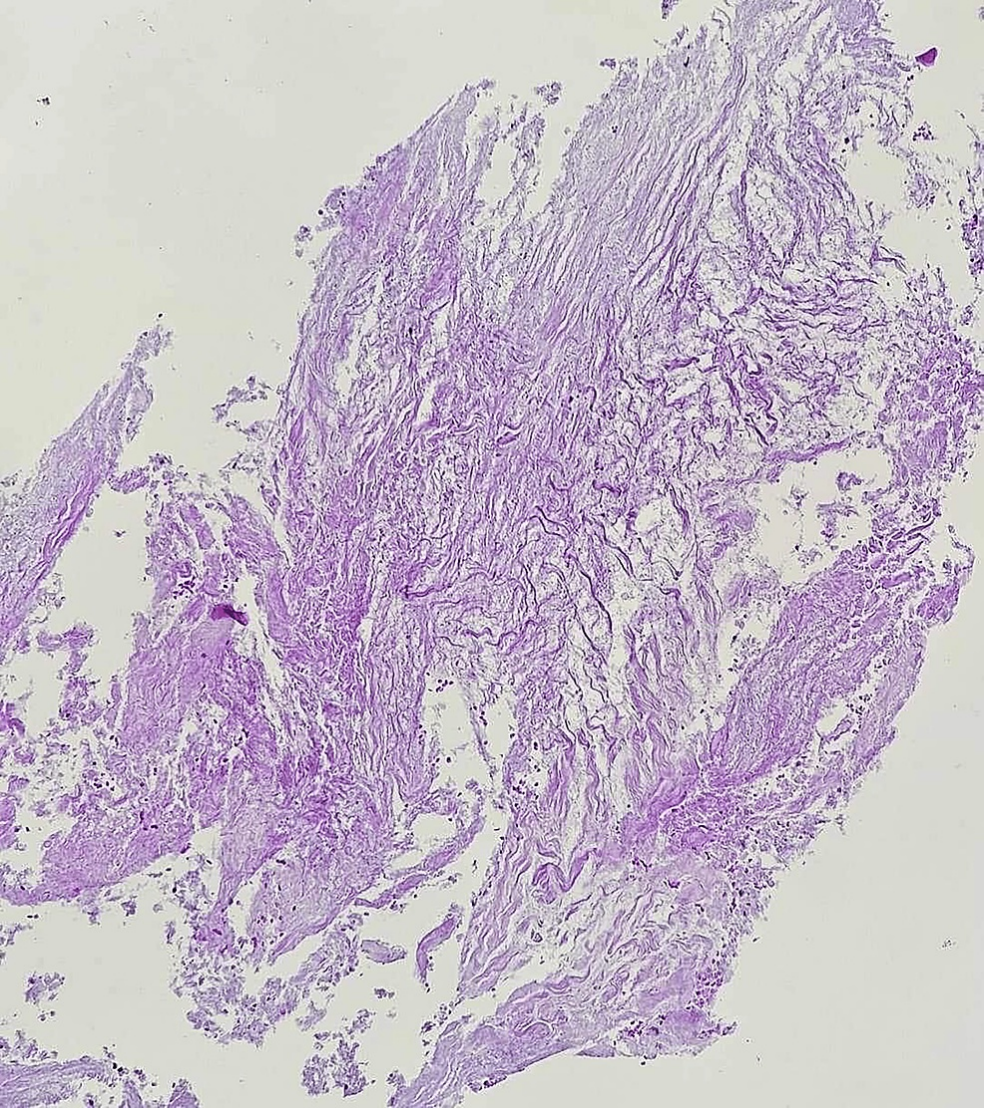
CT-guided biopsy of the nodule (August 10, 2023). CT, computed tomography
